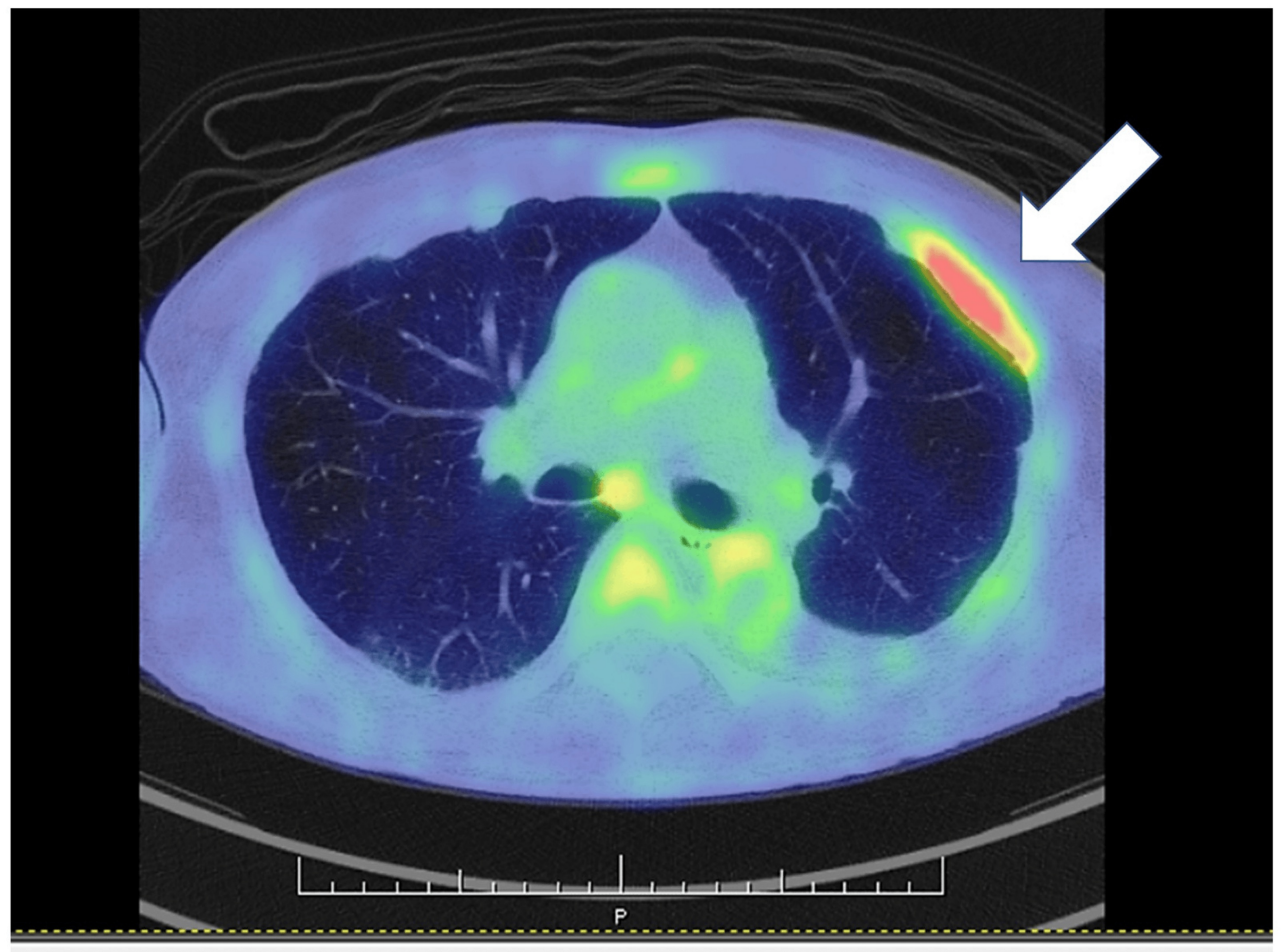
Positron emission tomography-computed tomography. Increased uptake (arrow), corresponding to the area of pleural thickening in the left third/fourth intercostal space (SUVmax: 4.89→5.77). SUVmax: maximum standardized uptake value
